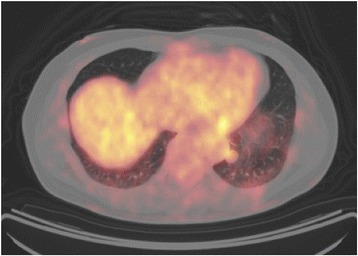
^18^F-fluorodeoxyglucose positron emission tomography (FDG-PET) revealed a nodular lesion with no abnormal uptake of the tracer
